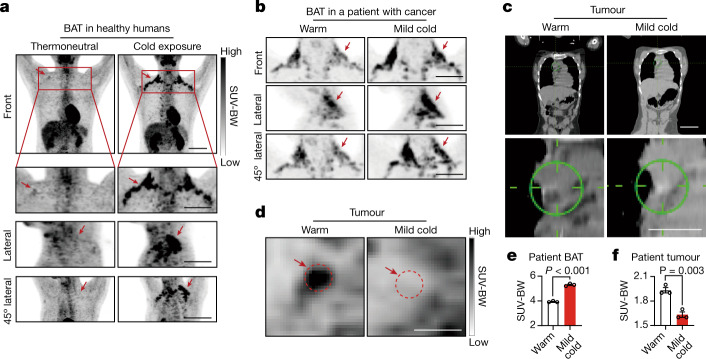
Activation of BAT by exposure to mild cold in healthy individuals and a patient with cancer. a, Representative PET images of ^18^F-FDG uptake in healthy male volunteers under thermoneutral (28 °C) and cold exposure (16 °C) conditions for 2 weeks. *n* = 3 individuals. Scale bars, 100 mm. b, Representative PET images of ^18^F-FDG uptake in the bilateral areas of supraclavicular, cervical and parasternal regions in a patient with Hodgkin’s lymphoma under warm and mild cold conditions. Scale bars, 100 mm. c, Representative images by CT scanning in the tumour region under warm conditions and exposure to mild cold. Scale bars, 100 mm (top) and 50 mm (bottom). d, PET images of ^18^F-FDG uptake of the tumour region under warm and mild cold conditions. Scale bars, 50 mm. Arrows indicate respective positive signals. e, Quantification of SUV-BW positive signals shown in b. *n* = 3 separate scans. f, Quantification of SUV-BW positive signals shown in d. *n* = 3 separate scans. For e and f, data are mean ± s.e.m. Statistical analysis was performed using two-sided unpaired *t*-tests (e and f). Source data
